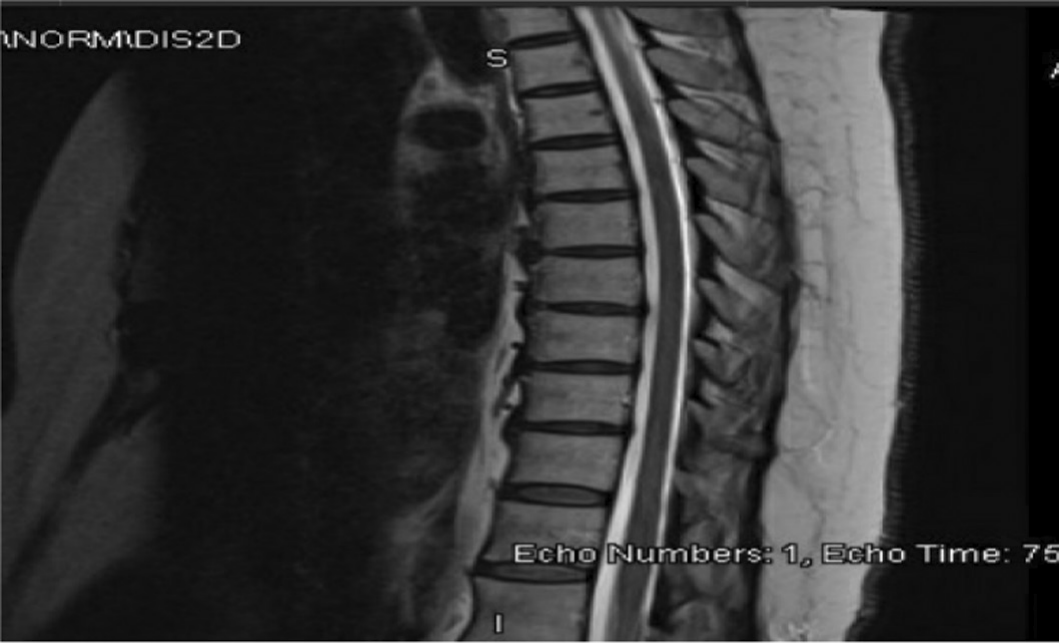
MRI of the thoracic spine
